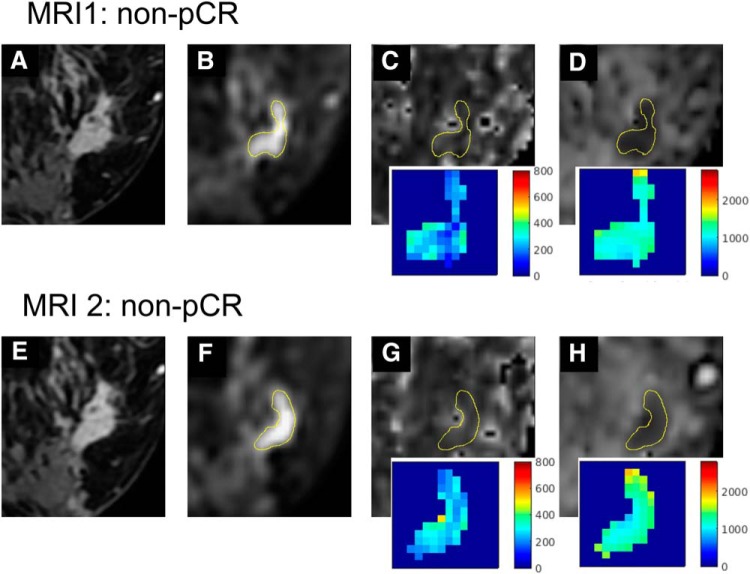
Example images from a patient with LABC who was categorized as non-pCR based on postsurgical pathology. DCE-MRI subtraction images (precontrast subtracted from early postcontrast) and DTI images (b = 600, FA map, and ADC map) are shown for the pretreatment time points (A, B, C, and D) and early treatment time points (E, F, G, and H). The tumor ROI is indicated by the contour line on the DTI b = 600 images and FA and ADC maps, and colorized maps of the FA and ADC values within the tumor ROI are also shown.
